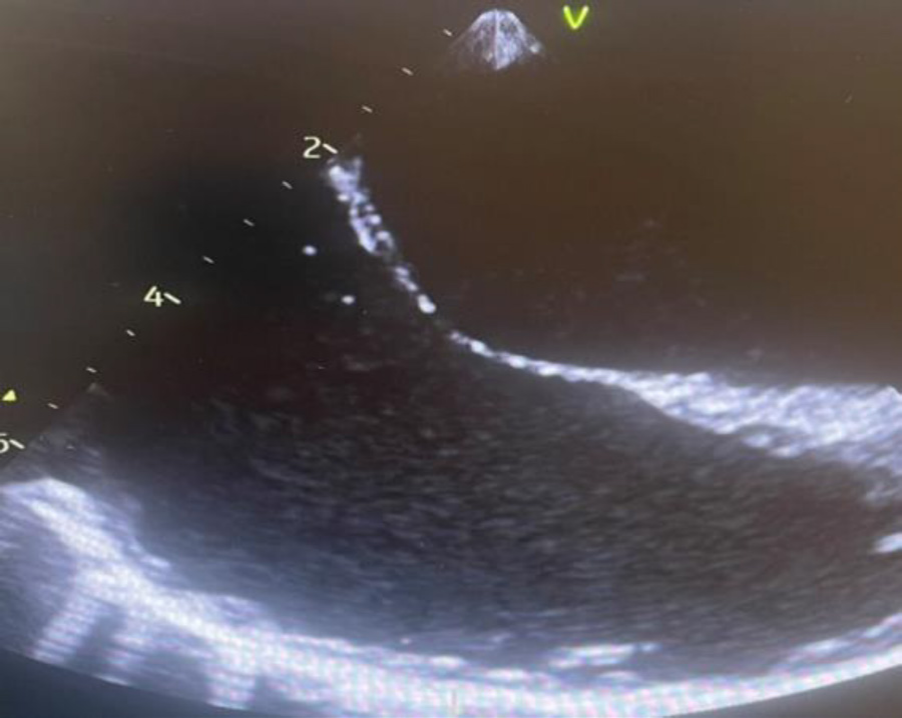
Normal transesophageal echocardiography after discharge.
